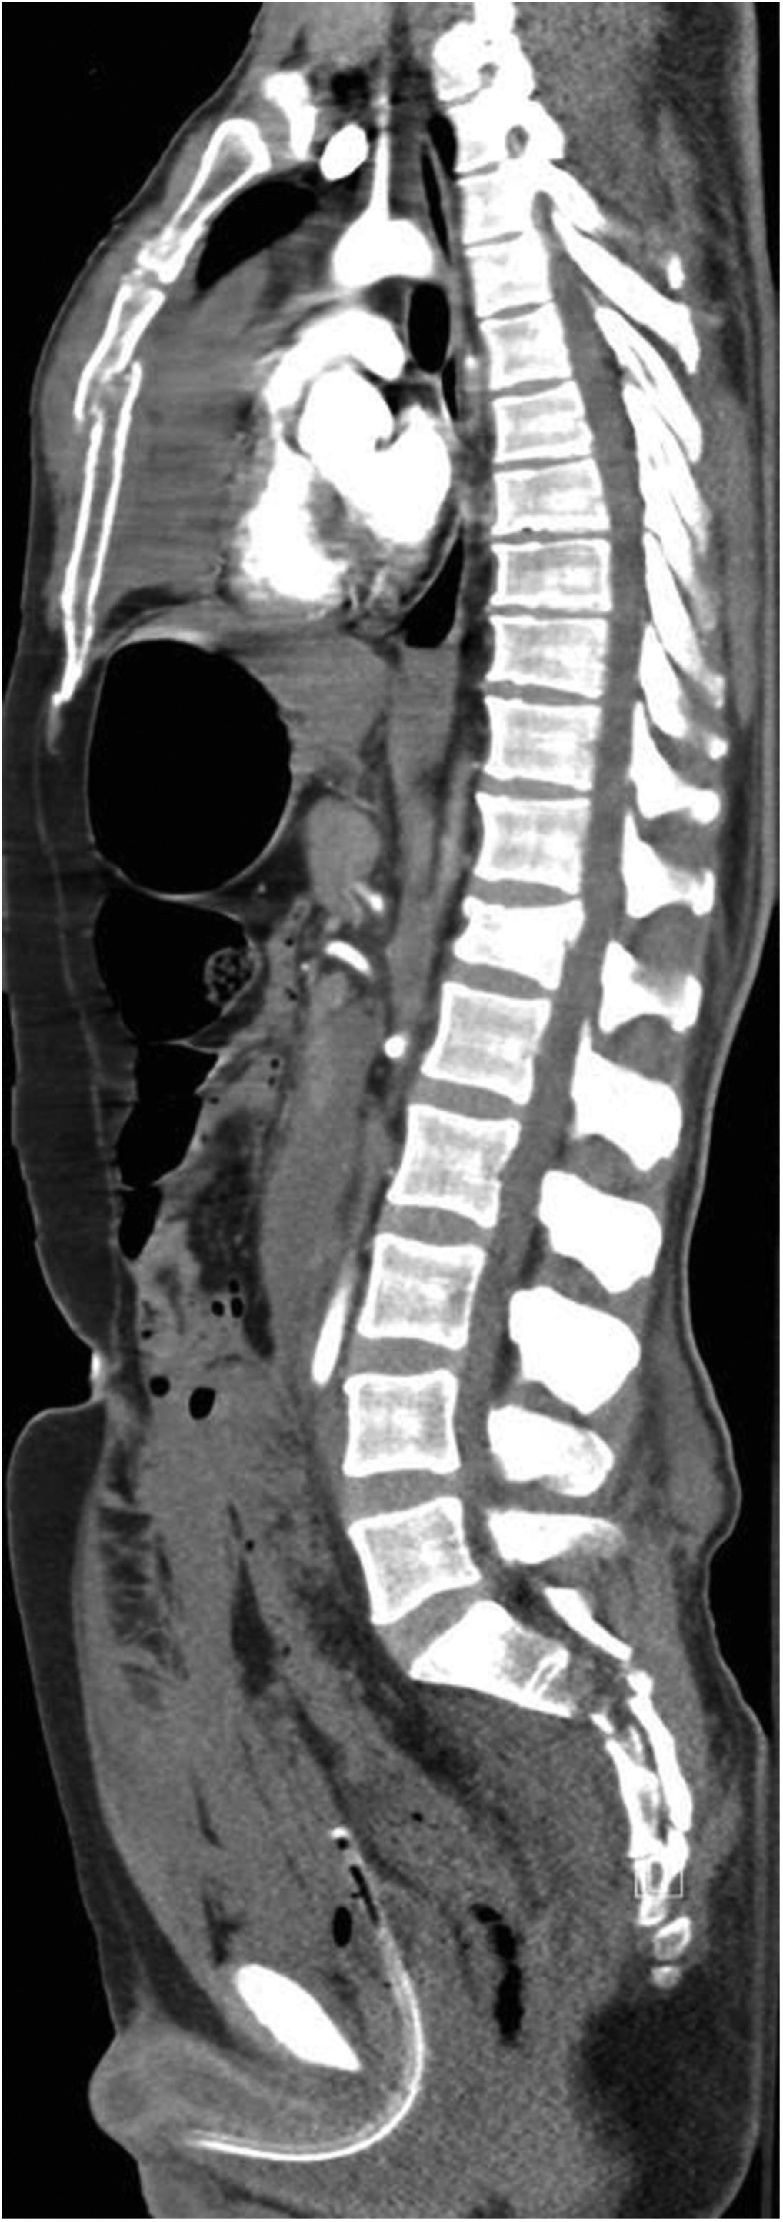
CT scan demonstrates a sternal fracture with Retrosternal hematoma.
